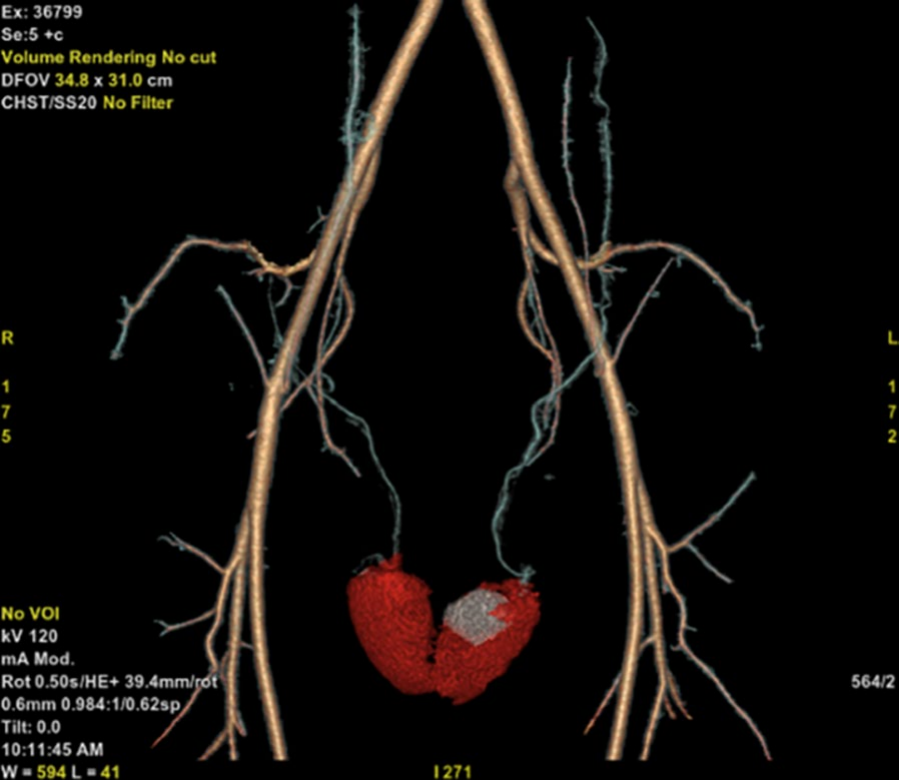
CT examination after infarction, three-dimensional reconstruction of CT showed a low-density area in the upper part of the left testis, with no obvious enhancement (Fig. 3)
